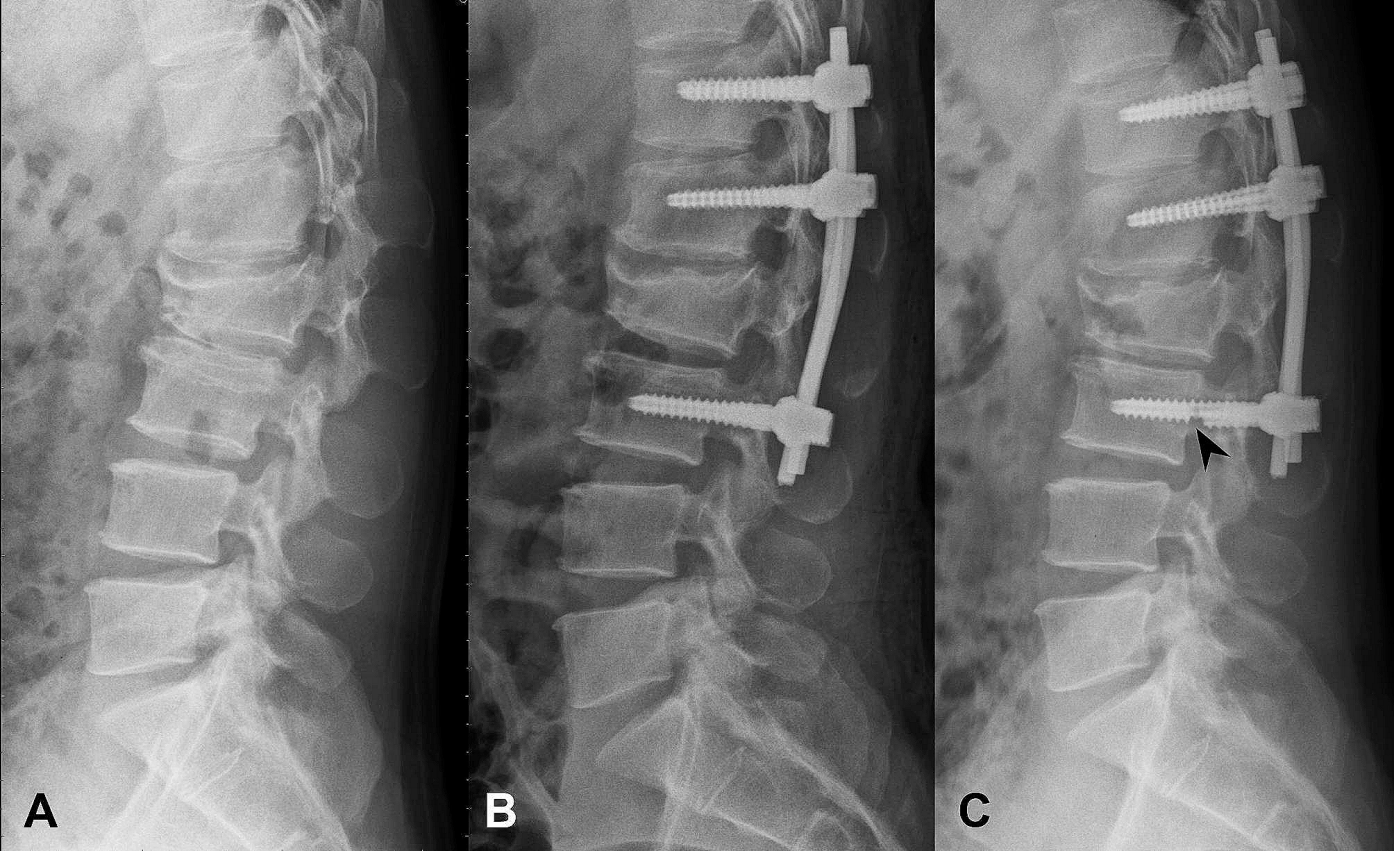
A 58-year-old female patient with an A3-type burst fracture at the L2 level and a McCormack load-sharing score of 5 underwent intermediate-segment pedicle screw fixation. A: Preoperative lateral spine radiography. B: Postoperative lateral spine radiography on day 1. C: A broken pedicle screw (arrowhead) seen in lateral spine radiography at the 6-month postoperative follow-up
